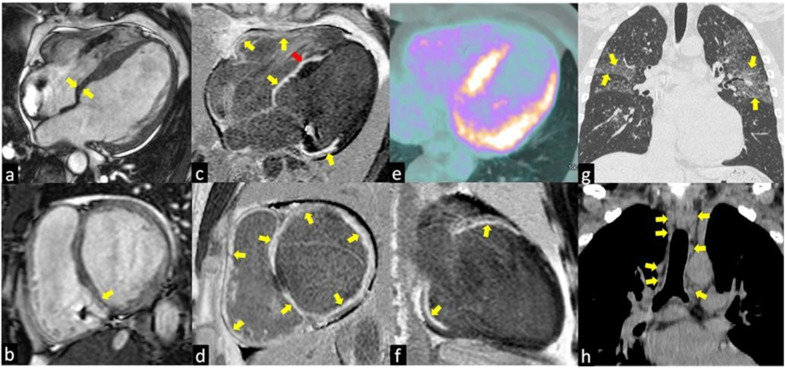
The images show a case of typical Cardiac Sarcoidosis characterized by the presence of LGE of all basal segments of both ventricles (yellow arrows in c,d,f) with the predominantly transmural distribution involving more than one coronary territory and the right ventricular side of the interventricular septum (red arrow in c). Bright blood cine sequences show the thinning of the basal septum (yellow arrows in a,b). Coronal computed tomography (CT) scans show the typical perilymphatic distribution of micronodules with upper lobe predilection (yellow arrows in g) and hilar and mediastinal bilateral lymphadenopathy (yellow arrows in h). The 18F-fluorodeoxyglucose positron emission tomography (e) revealed an increased uptake in the septal and lateral left ventricle myocardial segments in a patient with systemic sarcoidosis.
